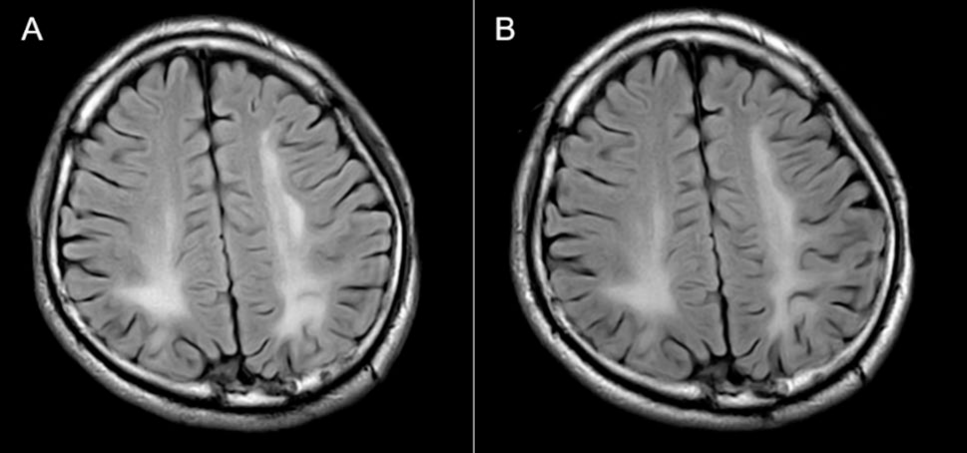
Post-treatment axial FLAIR (B) demonstrated persistence of the symmetrical supratentorial white-matter hyperintensities, with a slightly reduced extent compared with the pre-treatment axial FLAIR (A)
